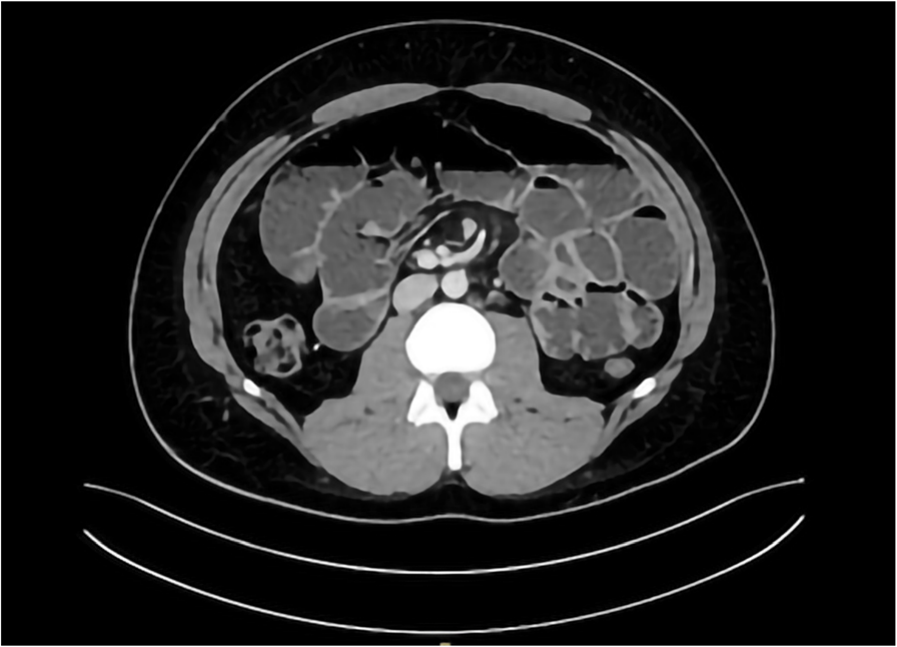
CT scan with intravenous contrast showing small bowel distension with air-fluid levels, and a reduced distal ileum diameter in the right iliac fossa
